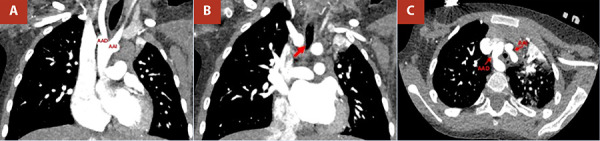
Computed tomography angiography of the chest. (A) Origin of the right and left aortic arches from the ascending aorta. (B) The right aortic arch produces extrinsic compression of the distal trachea (minimum diameter 3.4 mm) (red arrow). (C) Double right and left aortic arch forming a complete vascular ring around the trachea and oesophagus. RAA: right aortic arch. LAA: left aortic arch.
